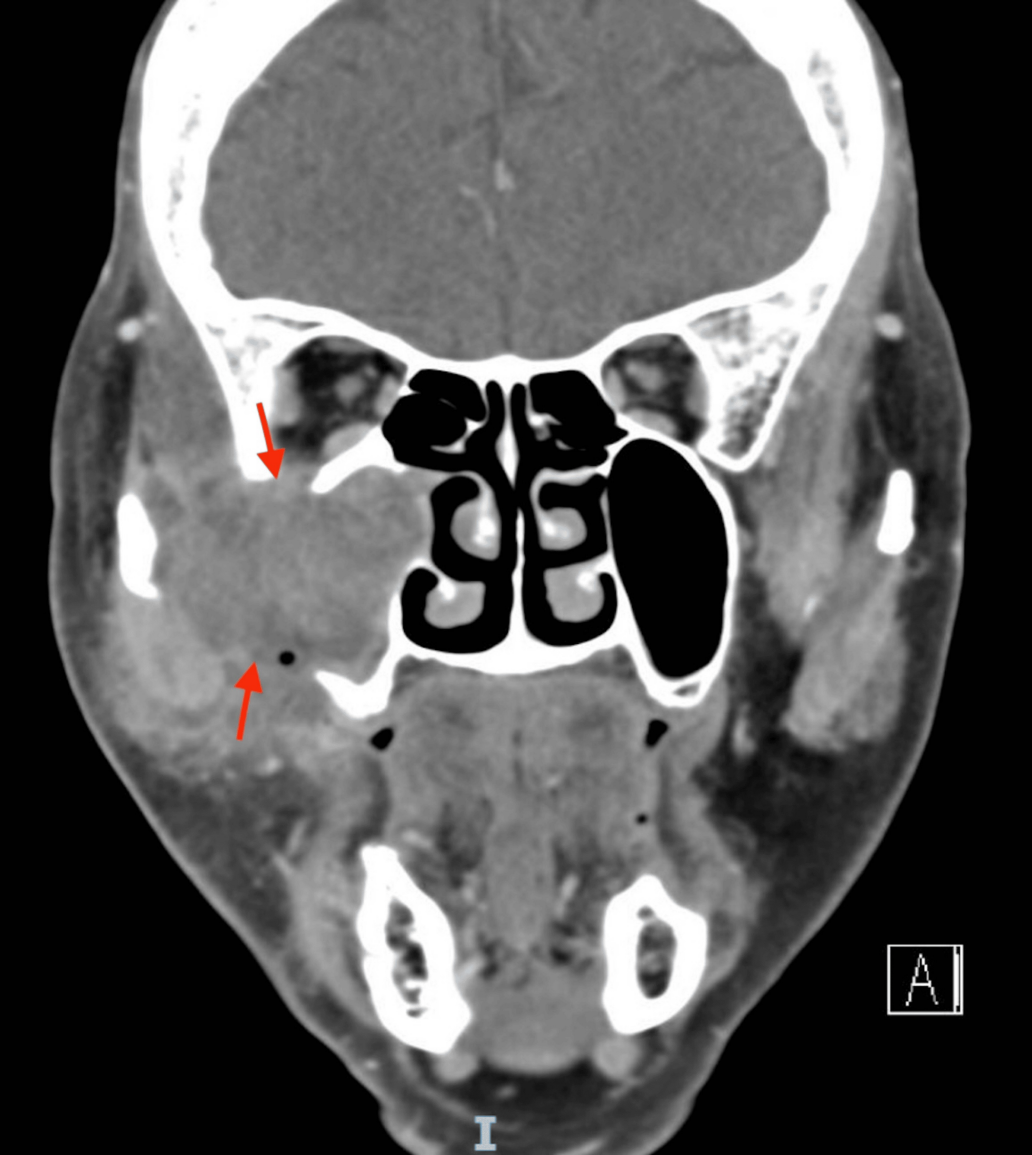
CT of the face depicting a heterogeneously enhancing soft tissue opacification with areas of rim enhancement, involving the right maxillary sinus, associated with the destruction of the anterior and lateral maxillary sinus with extension into the pre-antral fat, buccal and masticator spaces, and the floor of orbit erosion CT: computed tomography
